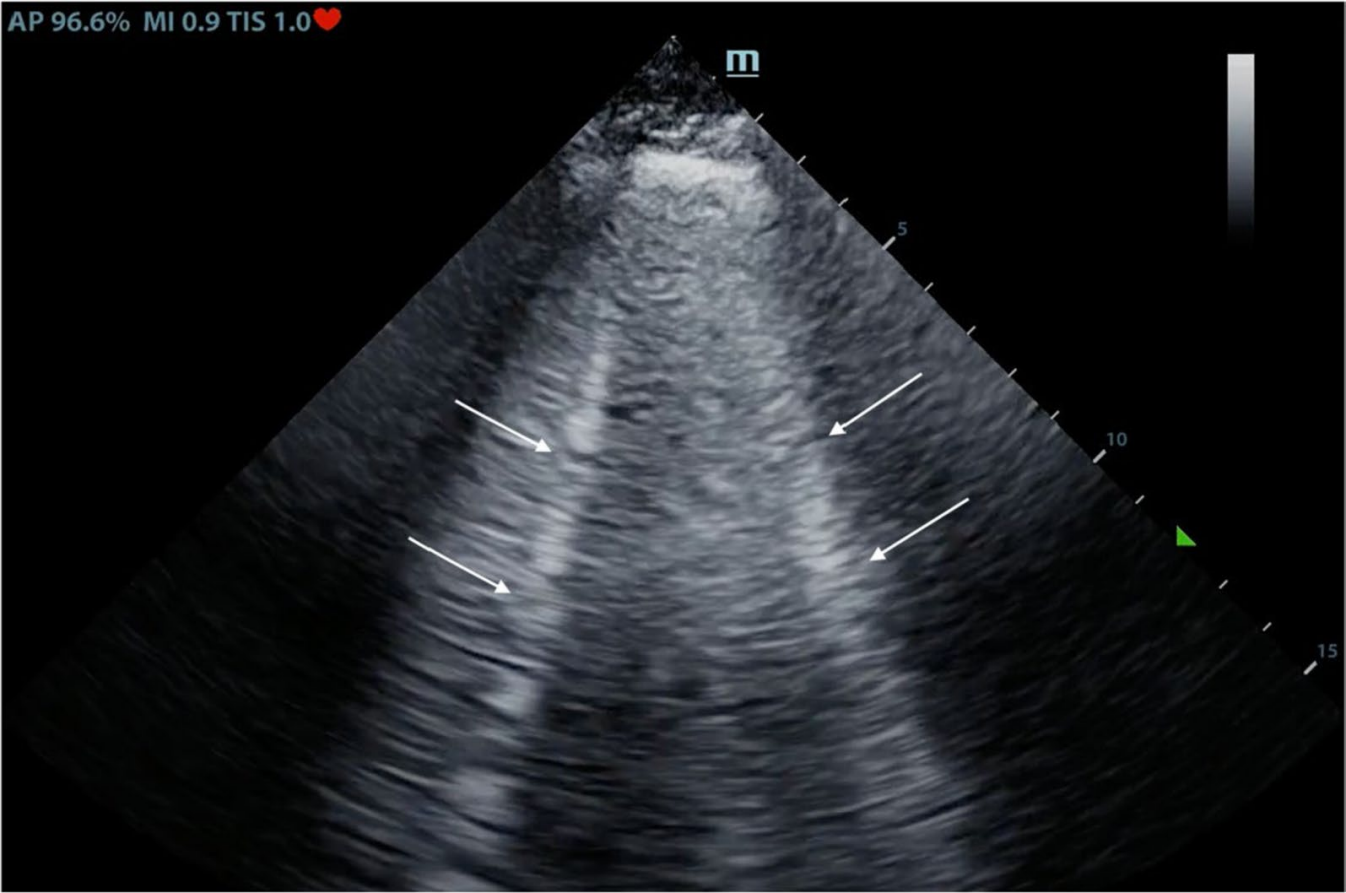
B-lines (white arrows) which are vertical hyperechoic lines that begin at the pleural line and extend all the way down to the bottom of the ultrasound screen
